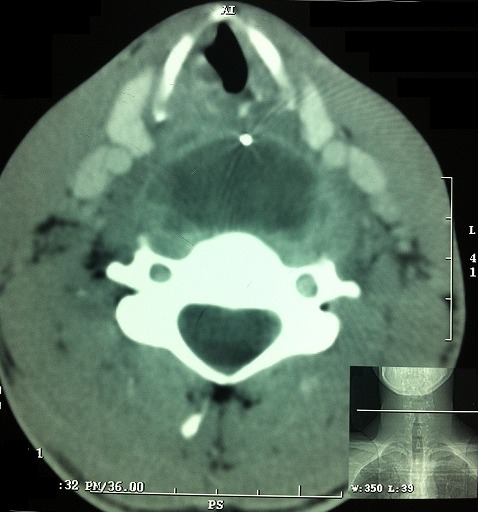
Cervical CT scan objectifying a retropharyngeal collection without discovertebral lysis and also confirming the presence of the foreign body
